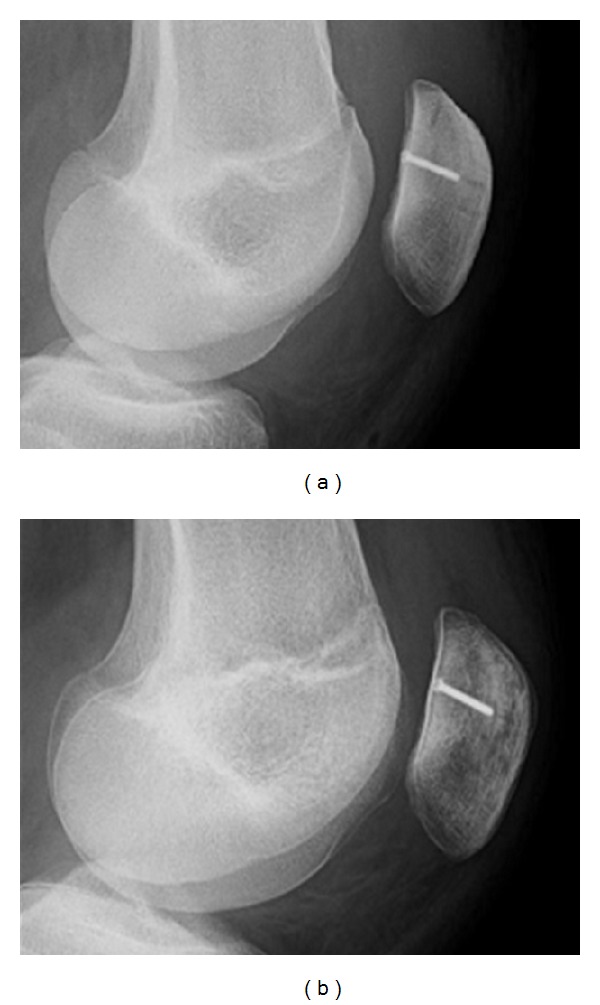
Serial axial radiographs of the patella after screw refixation of an osteochondral flake fracture in a 17-year-old patient. Followup imaging after operation (b) shows osteopenia with a subchondral predelication, this is in clear contrast to the immediately postoperative radiograph (a).
